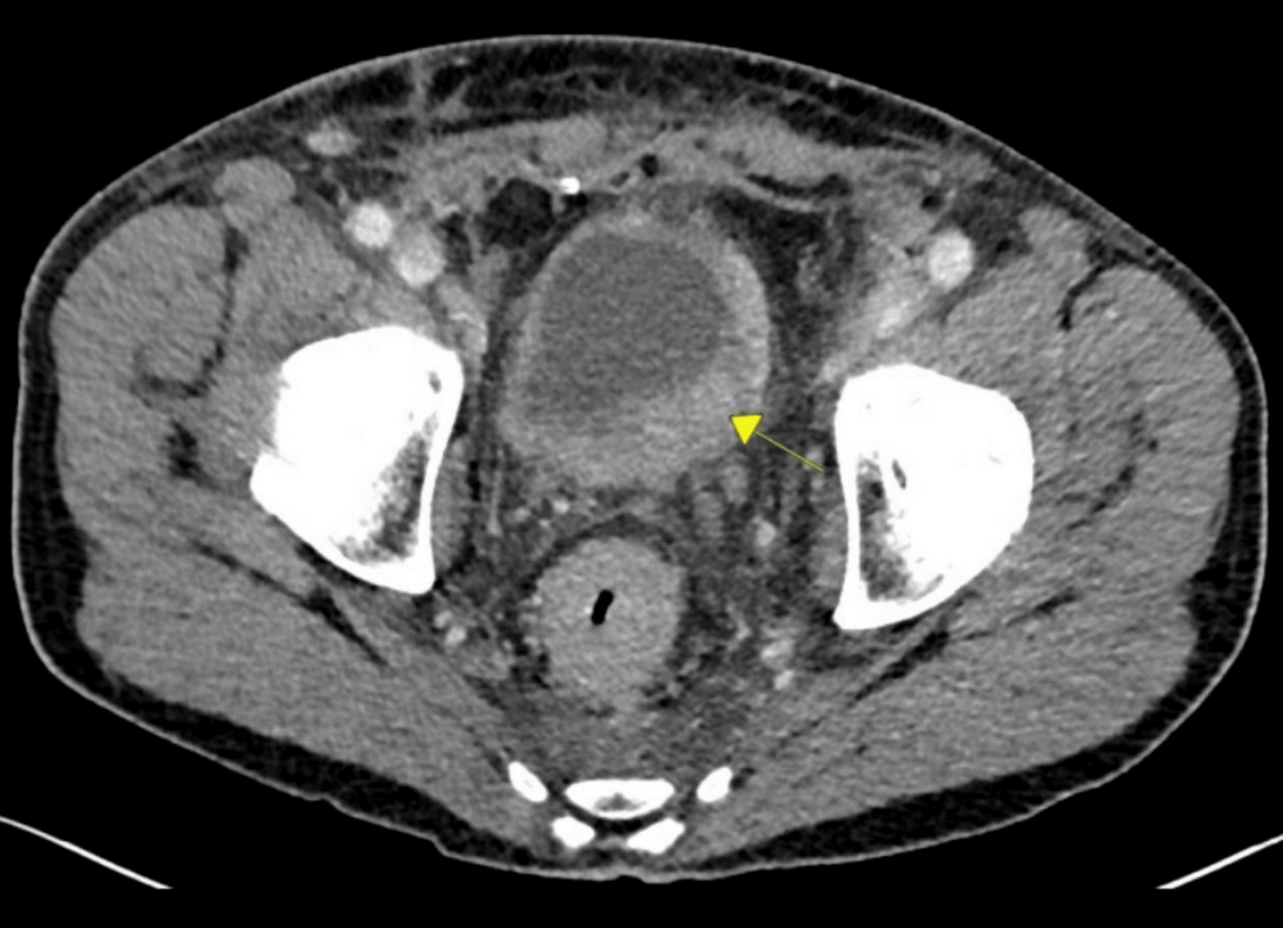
A CT abdomen and pelvis with contrast CT reveals irregular thickening of the bladder concerning for malignancy within the bladder (yellow arrow).
